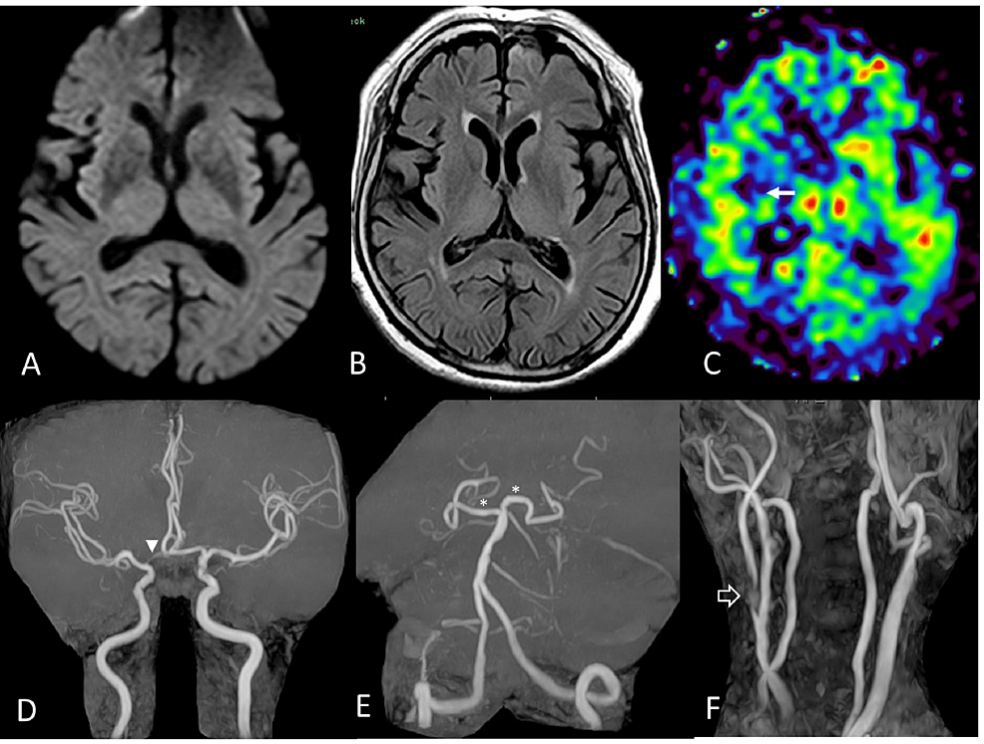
Preoperative magnetic resonance imaging (MRI) (A) MR diffusion-weighted imaging shows no acute infarction with the hyperintense signal. A metallic artifact of unknown origin is present in the left frontal region. (B) Fluid-attenuated inversion recovery (FLAIR) MRI shows no abnormalities around the right insular cortex. (C) Arterial-spin-labelling MRI shows decreased cerebral blood flow around the right insular cortex (arrow) compared to the left side. (D) There is no marked stenosis of the main intracranial artery. However, the A1 portion (arrow head) of the right anterior cerebral artery is narrow and nondominant. (E) The proximal portions (asterisk) of the bilateral posterior cerebral arteries are clearly demonstrated due to the posterior communicating arteries of the adult type. (F) Cervical MR angiography (MRA) shows severe stenosis with hyperintense unstable plaque at the origin of the right internal carotid artery (white arrow). There is no significant stenosis in the left internal carotid artery.
